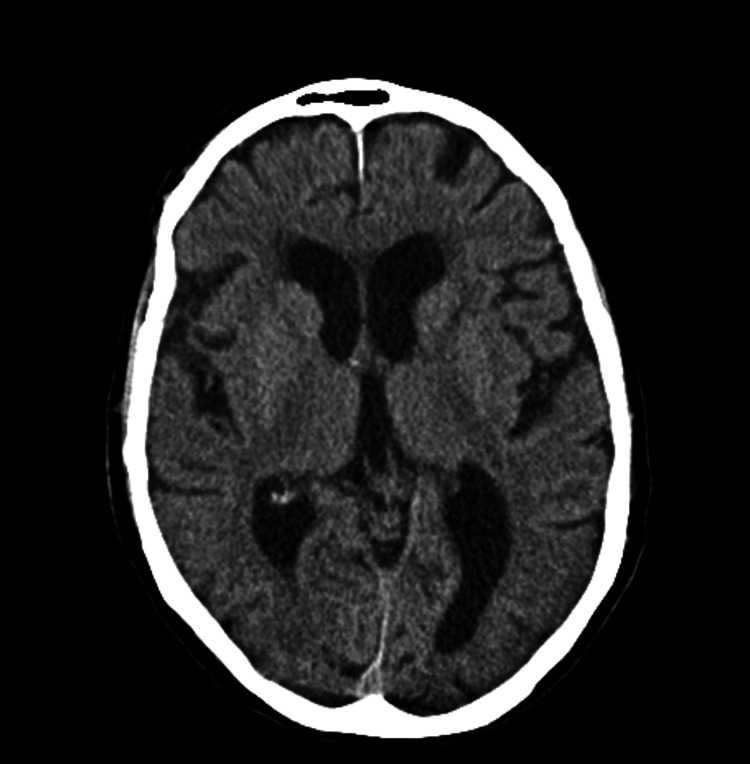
Computed tomography angiography of the cerebral vessels after the second event.
